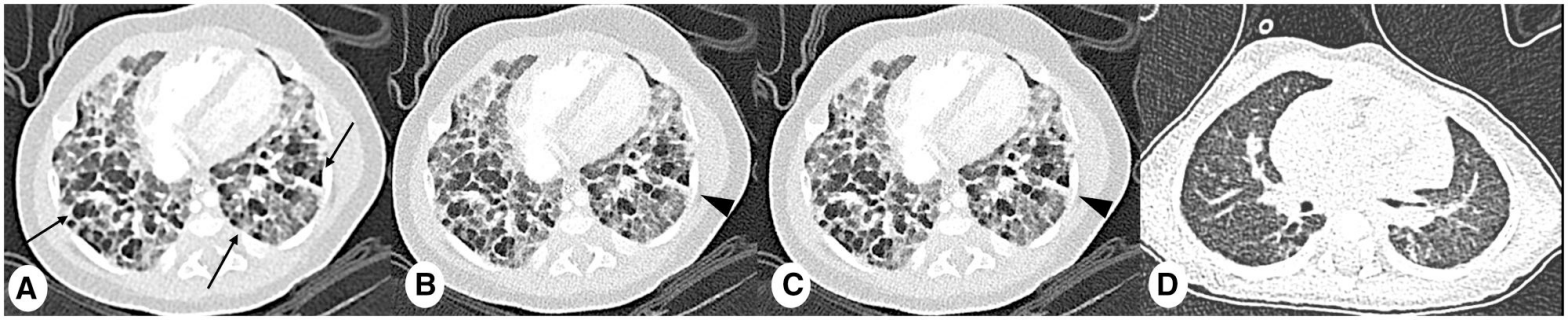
Comparison of image quality in infants with bronchopulmonary dysplasia (BPD). Axial PCD-CT images in 3 months old male with BPD, (A) slice thickness 1 mm, reconstruction kernel Bl56 and IR level 2, Q2, (B) slice thickness 1 mm, reconstruction kernel Bl64 and IR Q2, and (C) slice thickness 0.6 mm, reconstruction kernel Bl64 and IR Q2. (D) EID-CT coronal image (slice thickness 1 mm, reconstruction kernel Bl57 and IR 3). Note significant increase of sharpness and reduced noise from PCD-CT images, which facilitate assessment of typical BPD features, such as hypodense focal abnormalities (thin arrows) and linear fibrotic consolidations (arrowheads). Both scans are obtained with a dose-length product (DLP) of 4 mGy×cm.
